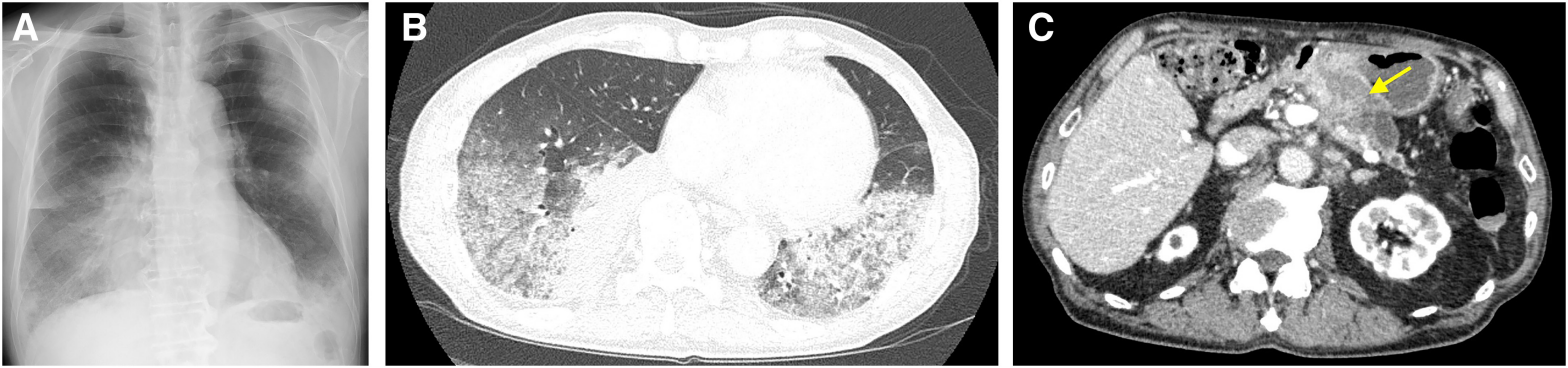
(A) Chest radiograph showing ground‐glass opacities and consolidations in both lung fields. (B) CT showing ground‐glass opacities and consolidations in both lung fields. (C) Contrast‐enhanced CT showing a pancreatic mass (an arrow).
